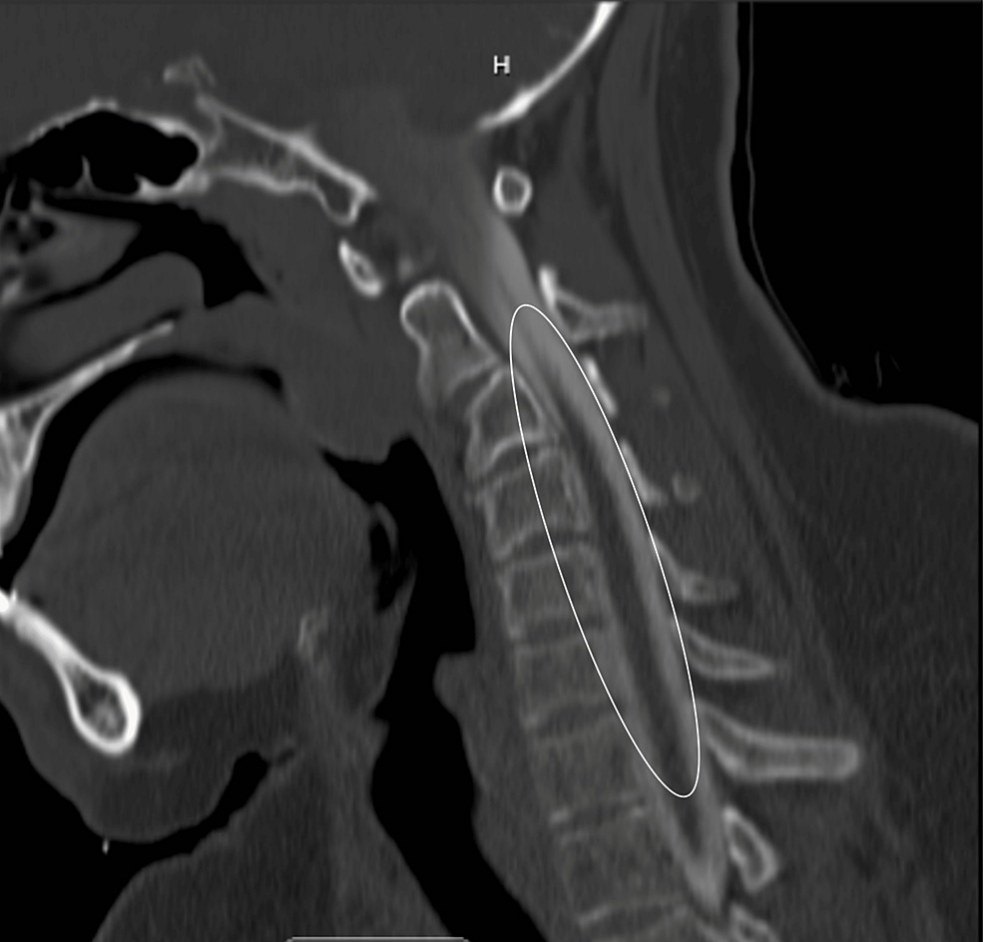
CT cervical myelogram with mild disc bulge present from C3-C7 with no foraminal stenosis
